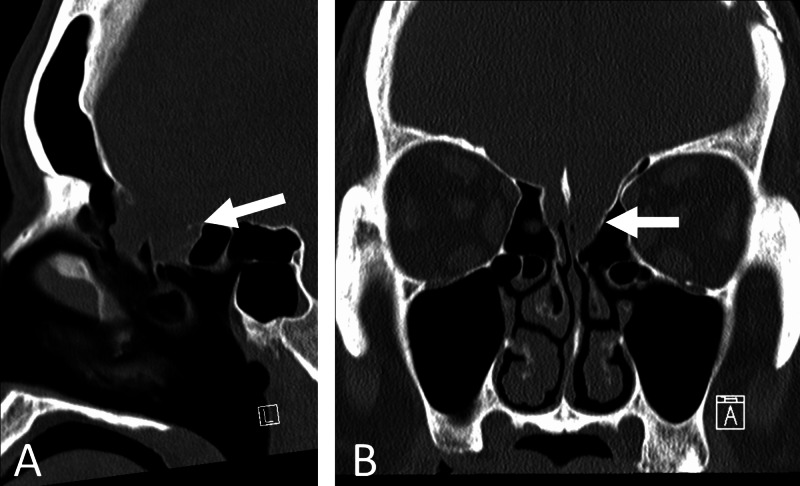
(A) Sagittal and (B) coronal bone windows of CT demonstrating bony defect in cribriform plate. CT, computed tomography
